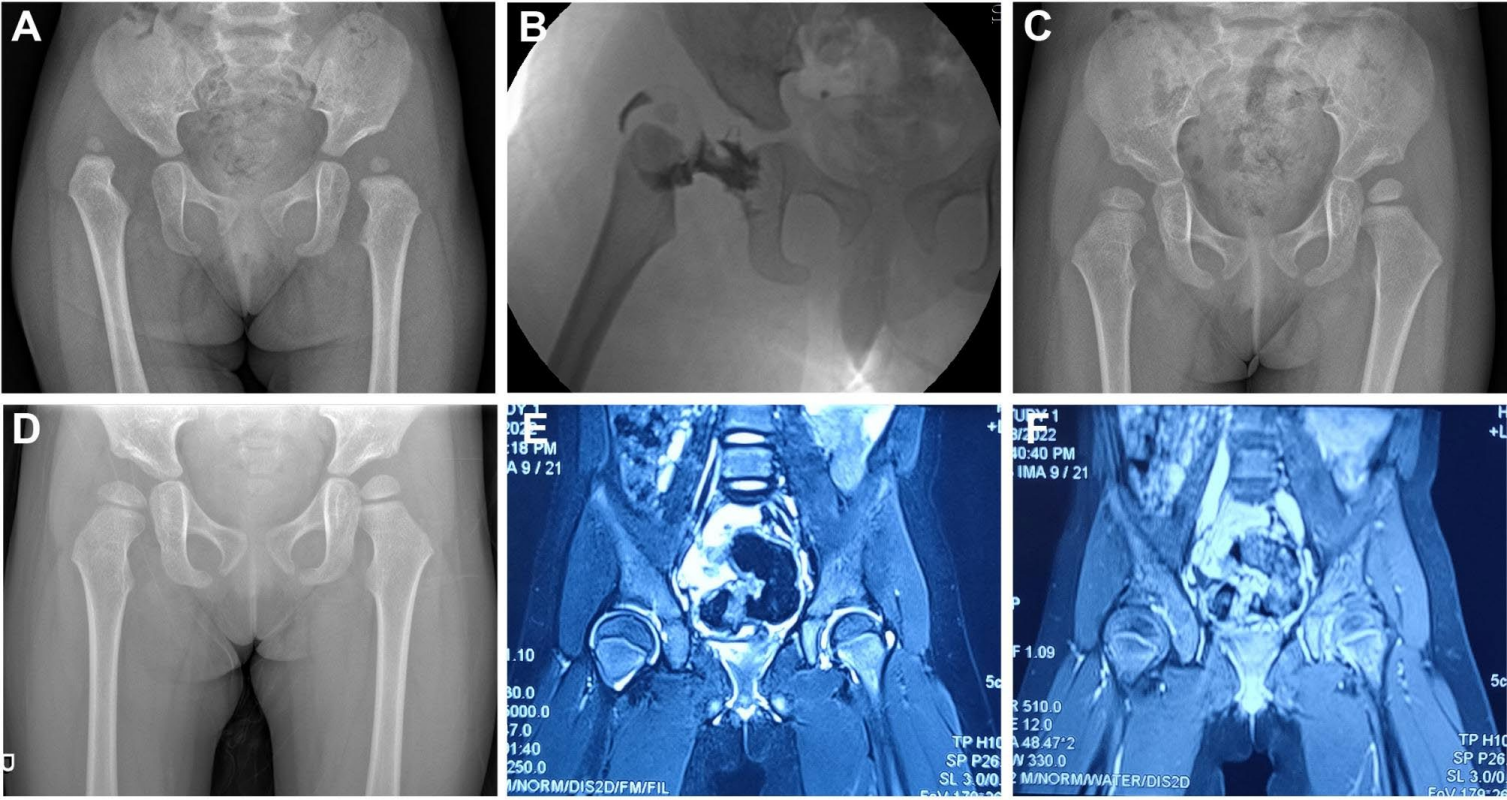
A. A 10-month-old female patient was admitted to the hospital due to inconsistency of bilateral breech lines, and was diagnosed as DDH. The preoperative acetabular index was 42.1°, IHDI was type IV; B. The pictures of arthrogram during operation; C. The follow-up of 14 months after operation showed that the reduction of the right hip joint was well restored, and the acetabular index was 29.2°, IHDI was type I; D. 2.5 years after surgery, X-ray showed that the right femoral head developed well, and the acetabulum was slightly dysplasia. E-F. 32 months after surgery, MRI showed good hip joint position and no ischemic necrosis of the femoral head
